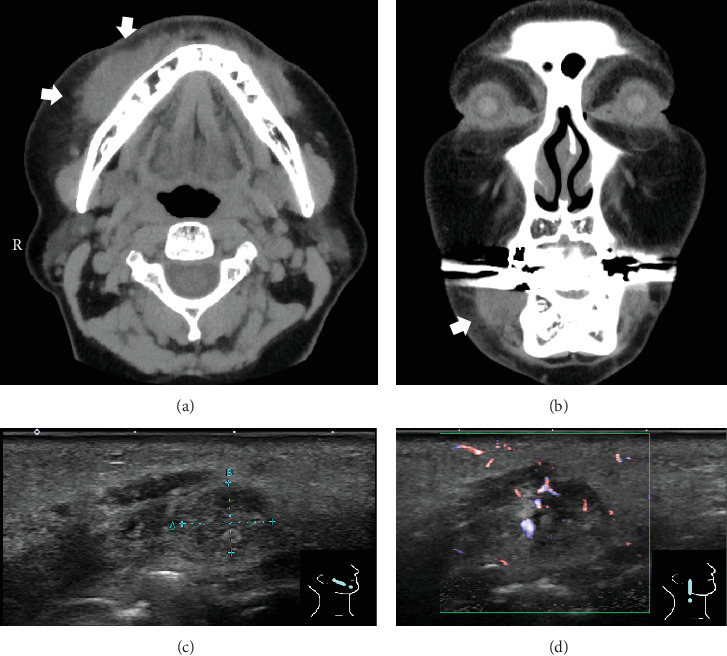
Clinical images of the patient at the first visit. (a, b) Computed tomography of the neck. A lesion equal in density to muscle was found under the right subcutaneous mandible (arrow). The tumour measured approximately 28 × 15 mm in thickness. There was an increased density of surrounding fatty tissue, suggesting an inflammatory mass ((a) axial image; (b) coronal image). (c, d) Findings of ultrasound examination. A 9 × 7-mm lesion was detected in the subcutaneous tissue of the right buccal area, continuous with the muscular layer. The border was slightly indistinct and oval in shape with heterogeneous hypoechoic internalization. Doppler imaging shows a penetrating vascular form (arterial waveform).
